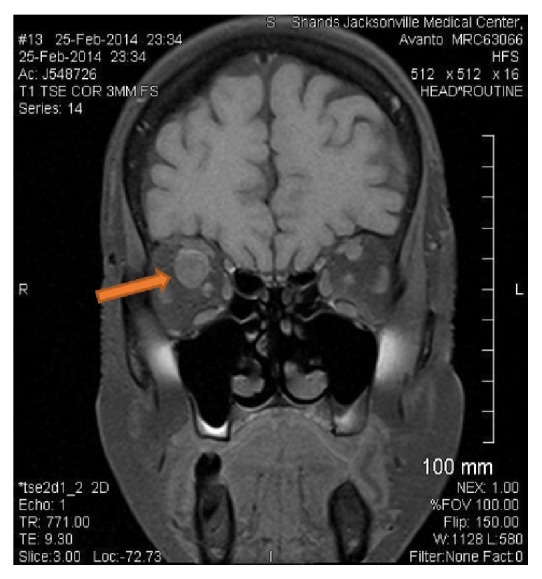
Brain MRI. Coronal section (T1) at initial presentation. The arrow is pointing to right SOV thrombosis.
